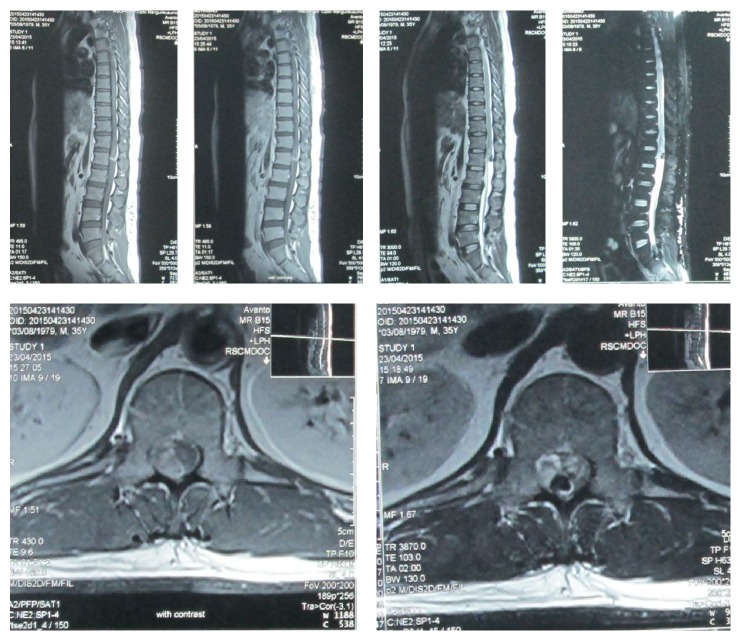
Sagittal and axial view of T1 and T1 with contrast-weighted images showing intradural intramedullary hyperintense signal at L1 level of spinal cord. Sagittal and axial T2-weighted images showing isointense signal of the lesion (taken on 23 June 2015).
